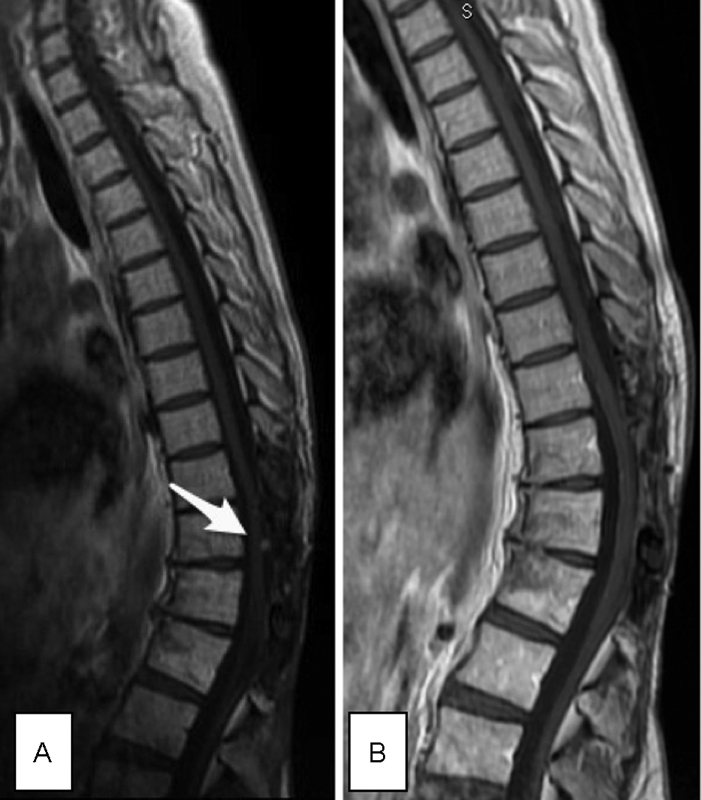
Third recurrence of the tumor in 2014 in MR imaging. (
